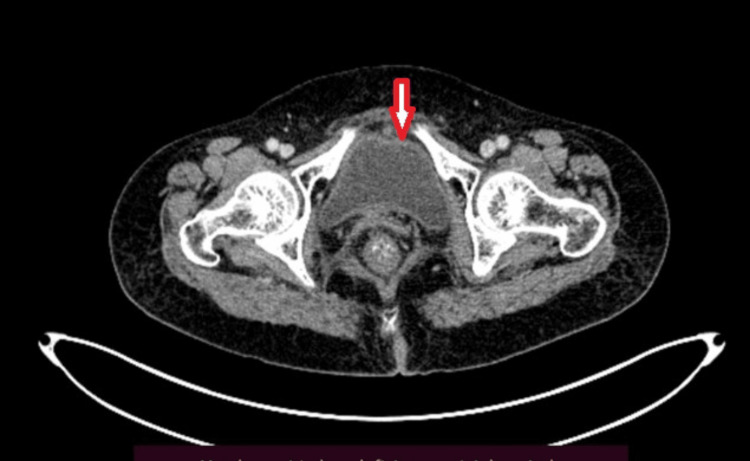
The CT image of the uterus in Case 2 shows an irregular, thickened uterus.
